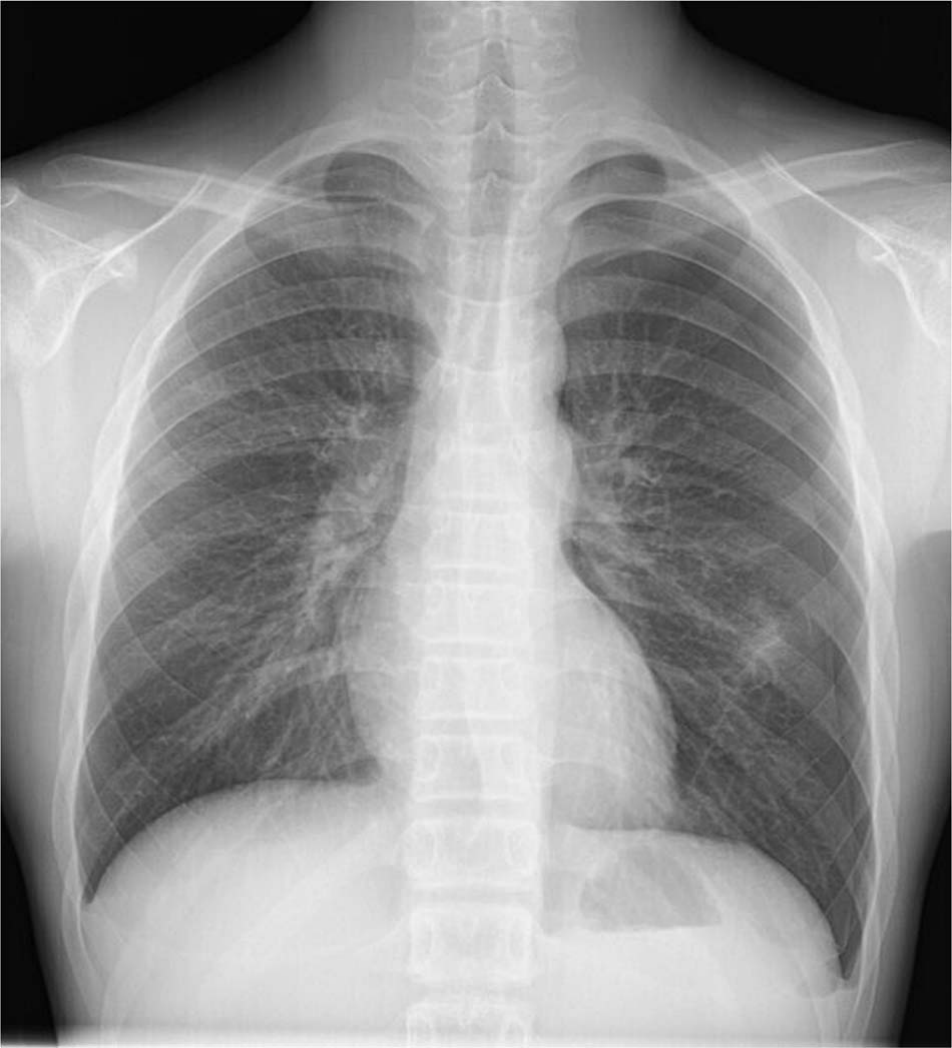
Chest radiography showing a left pneumothorax and an opacity in the left middle lung field.
